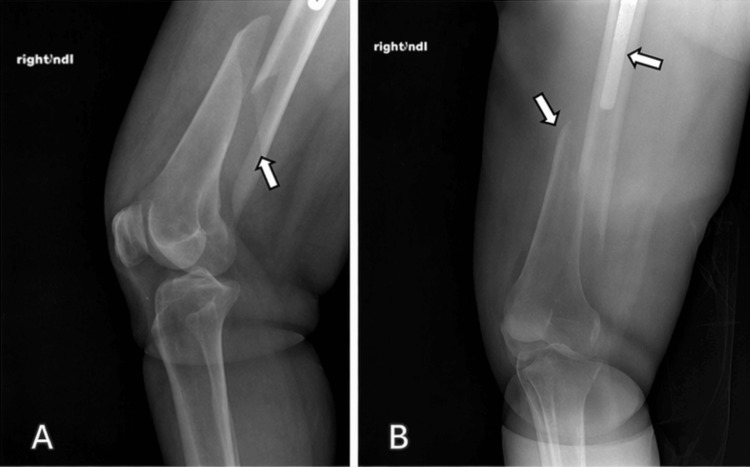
Anteroposterior (A) and lateral (B) preoperative radiograph demonstrating a displaced spiral fracture involving the distal femoral diaphysis consistent with Vancouver B1 periprosthetic fracture classification
